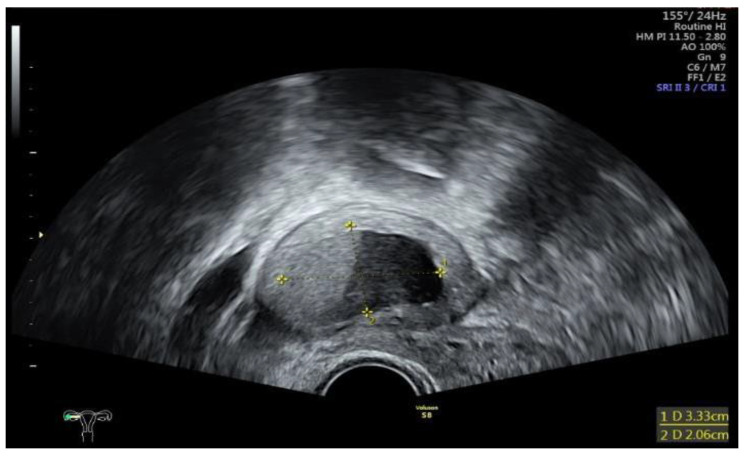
Sonographic display of a ruptured endometrioma of the right ovary in a 28-year-old woman with sudden onset of abdominal pain.
